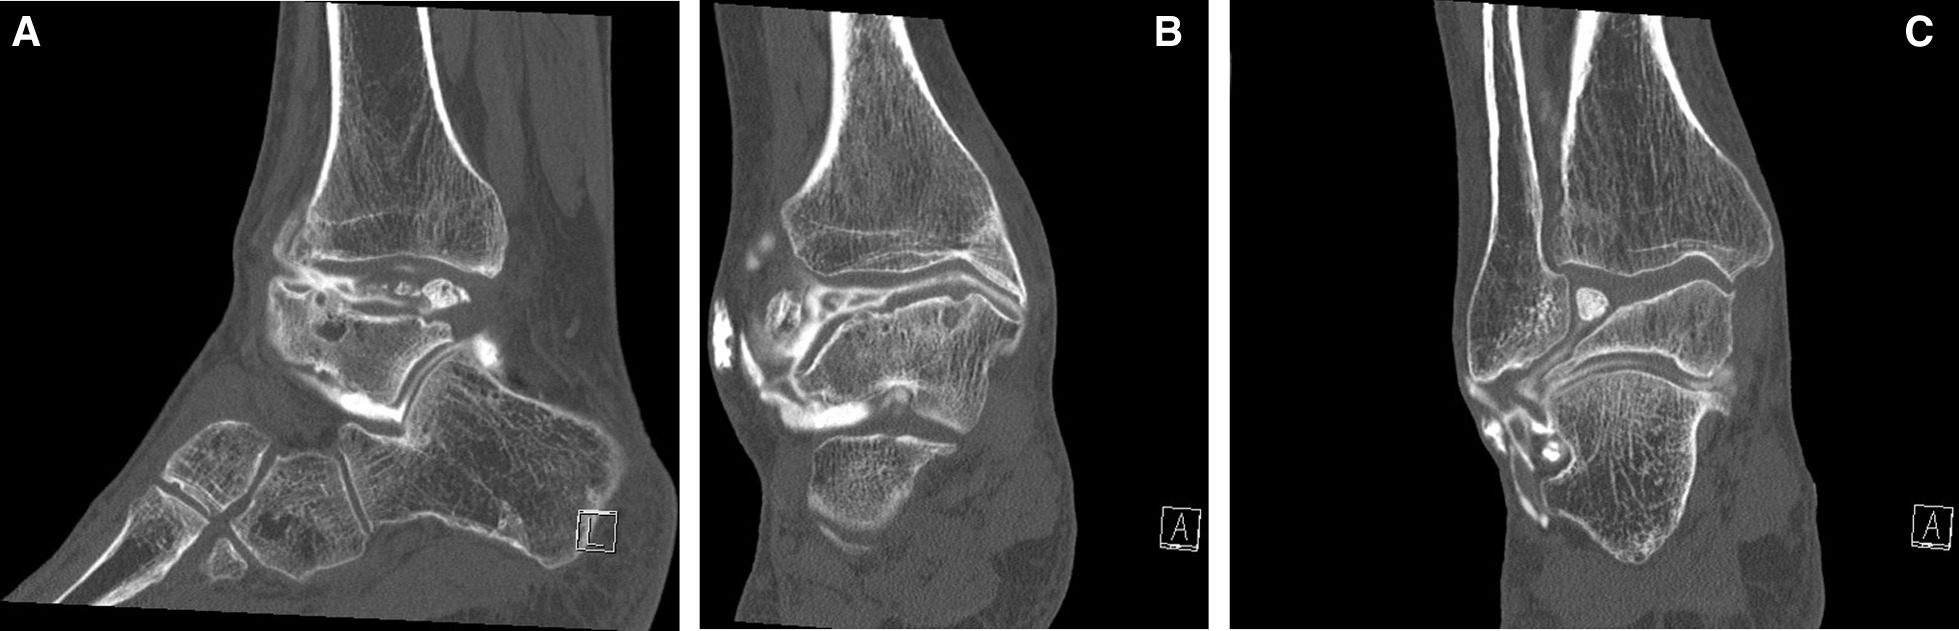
A Computed tomographic arthrography (sagittal image) of the ankle shows collapse of the talar dome, subchondral cysts, anterior fibrous-like tissues, and numerous posterior accessory ossicles. B Computed tomographic arthrography (frontal image) of the ankle shows subchondral talar cysts, meniscoid lesions, and diffuse intraarticular fibrosis. C Computed tomographic arthrography (frontal image) of the ankle shows an anterior intraarticular ossicle.
